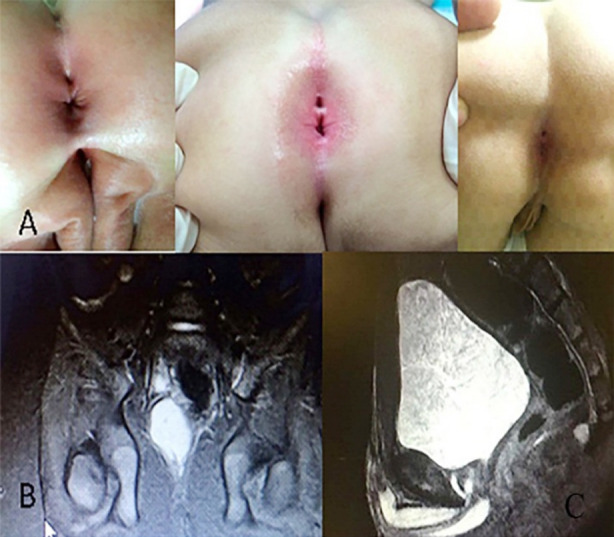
The perianal inspection demonstrates additional openings posterior to the anal orifice (A). Sagittal T1 weighted pelvic MRI demonstrates the presacral mass in patient 3 (B) and 4 (C).
